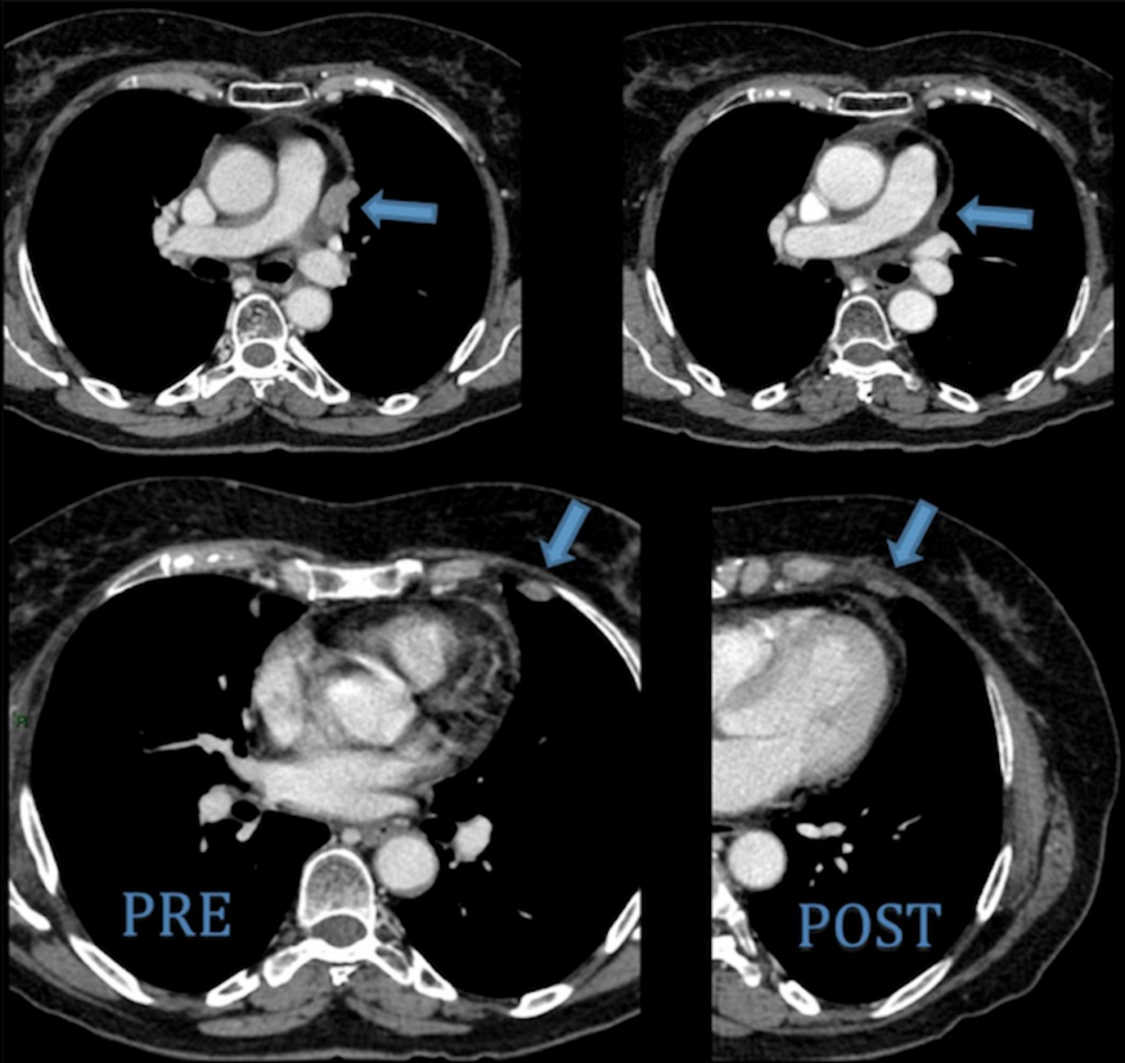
CT scan of May 2016 A significant reduction of pleural and pericardial implants is observed. The comparison between previous (PRE) and posterior (POST) CT to sunitinib treatment is shown. CT: computed tomography
